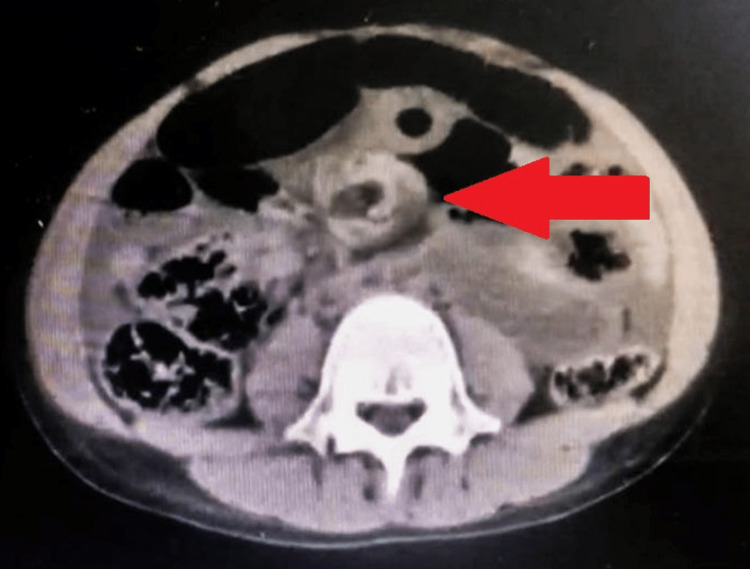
Abdominal CT scan Abdominal CT scan without contrast showing "whirl signs" at the level of the superior mesenteric artery, suggestive of midgut volvulus
